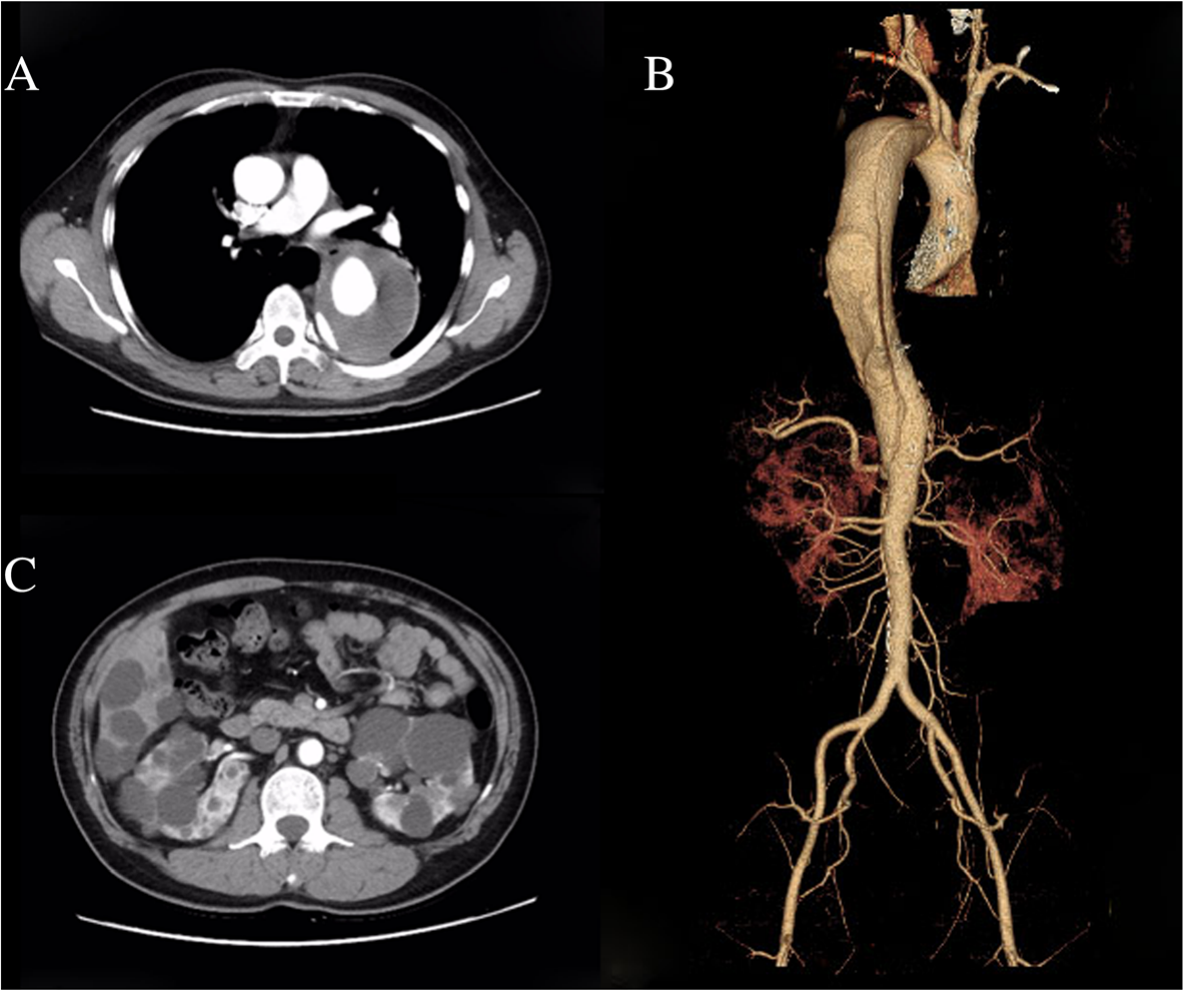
Radiographic findings of the proband. a Multi-slice computed tomography shows dissection aneurysm measuring 8.5 cm in diameter. b 3D–reconstructed computed tomography angiogram shows a Stanford B aortic dissection. c Multi-slice computed tomography shows multiple cysts in the liver and bilateral polycystic kidneys
